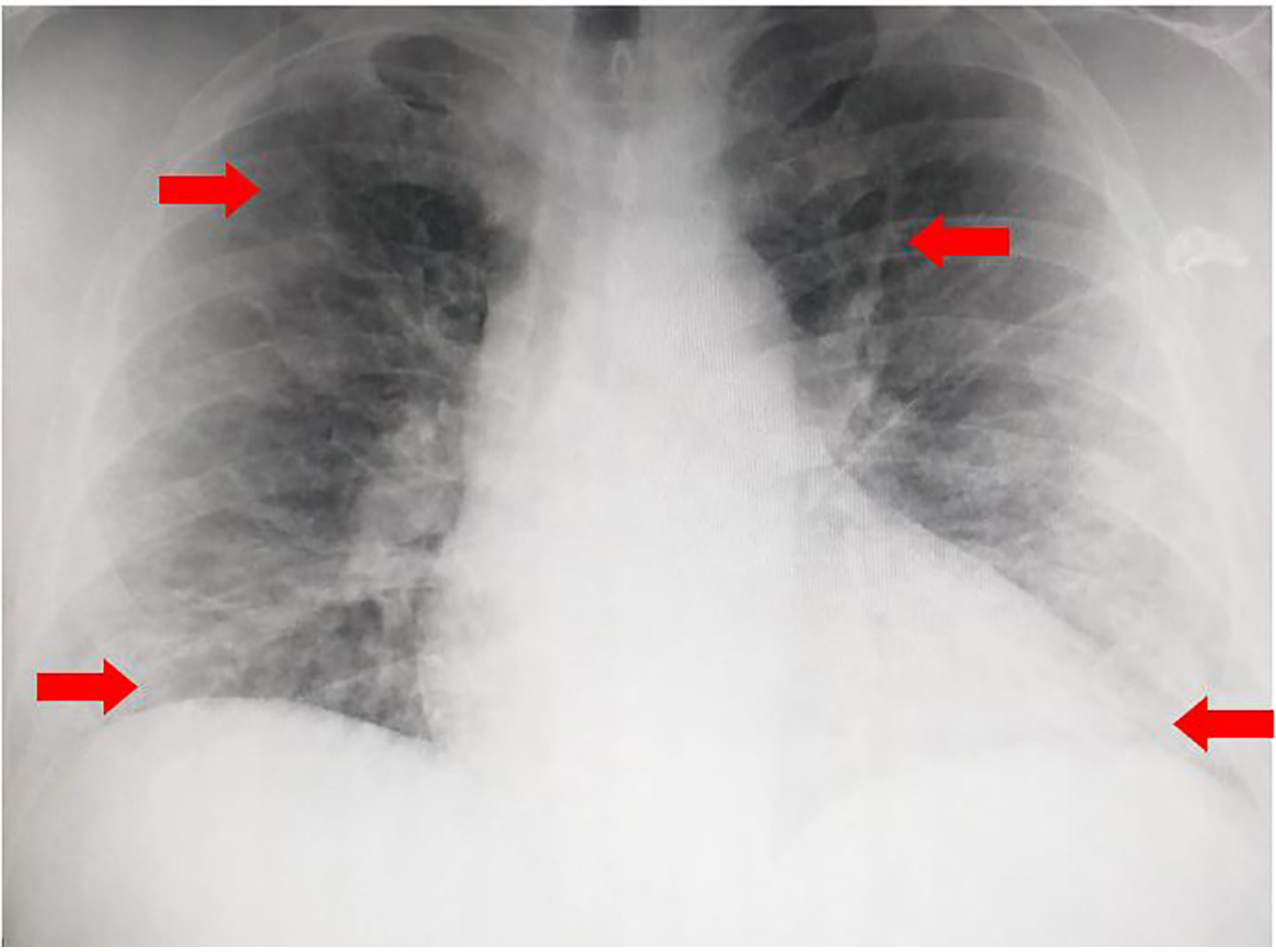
Initial chest radiograph demonstrating mild pulmonary vascular congestion with mild, hazy bilateral airspace disease, likely secondary to alveolar edema or aspiration (arrows).
